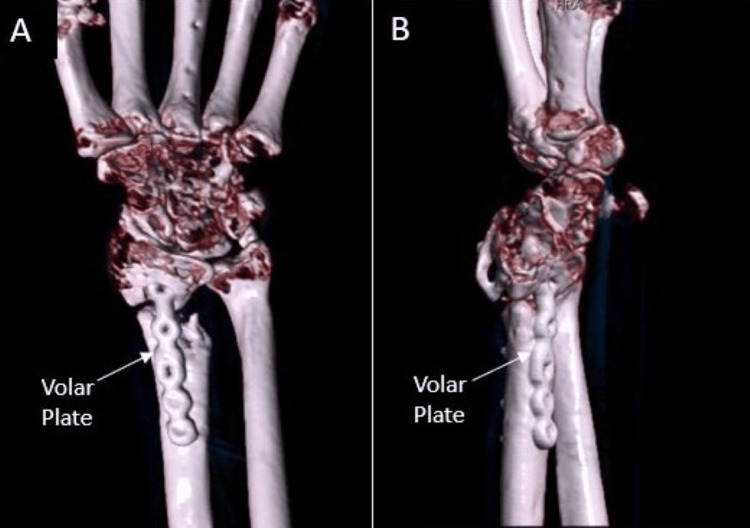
Repeat left wrist CT films done three months after DSP plate removal demonstrated interval callus formation with the hardware positioned appropriately and no violation of the radiocarpal joint A/B: 3D CT reconstructions of left wrist
